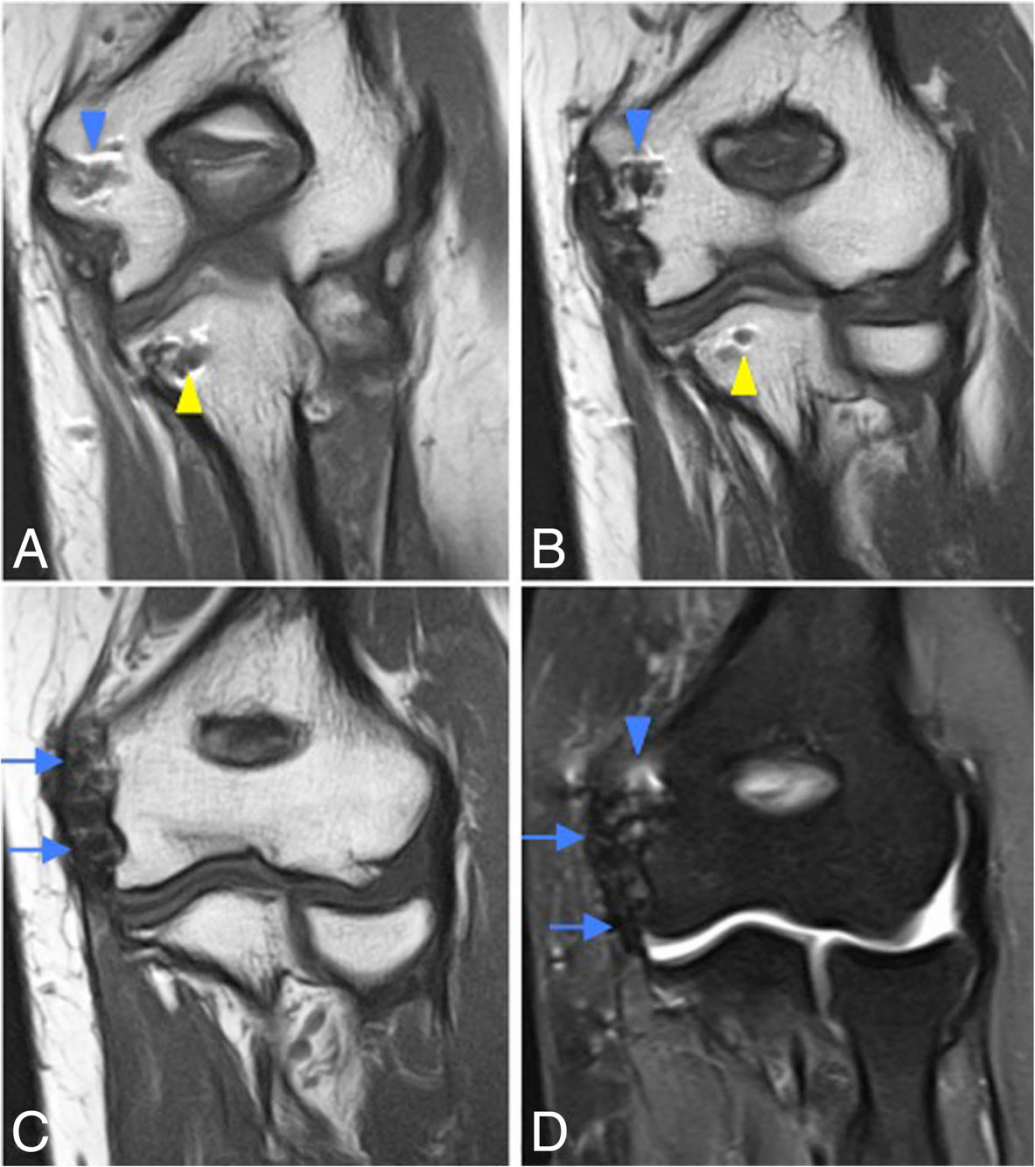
Normal appearance of reconstructed medial collateral ligament (docking technique). Consecutive coronal T1-weighted MRI (a–c) and coronal FS PD-weighted MRI (d). The intact graft (blue arrows) extends from humeral tunnel (blue arrowheads) to ulnar tunnel (yellow arrowheads)
